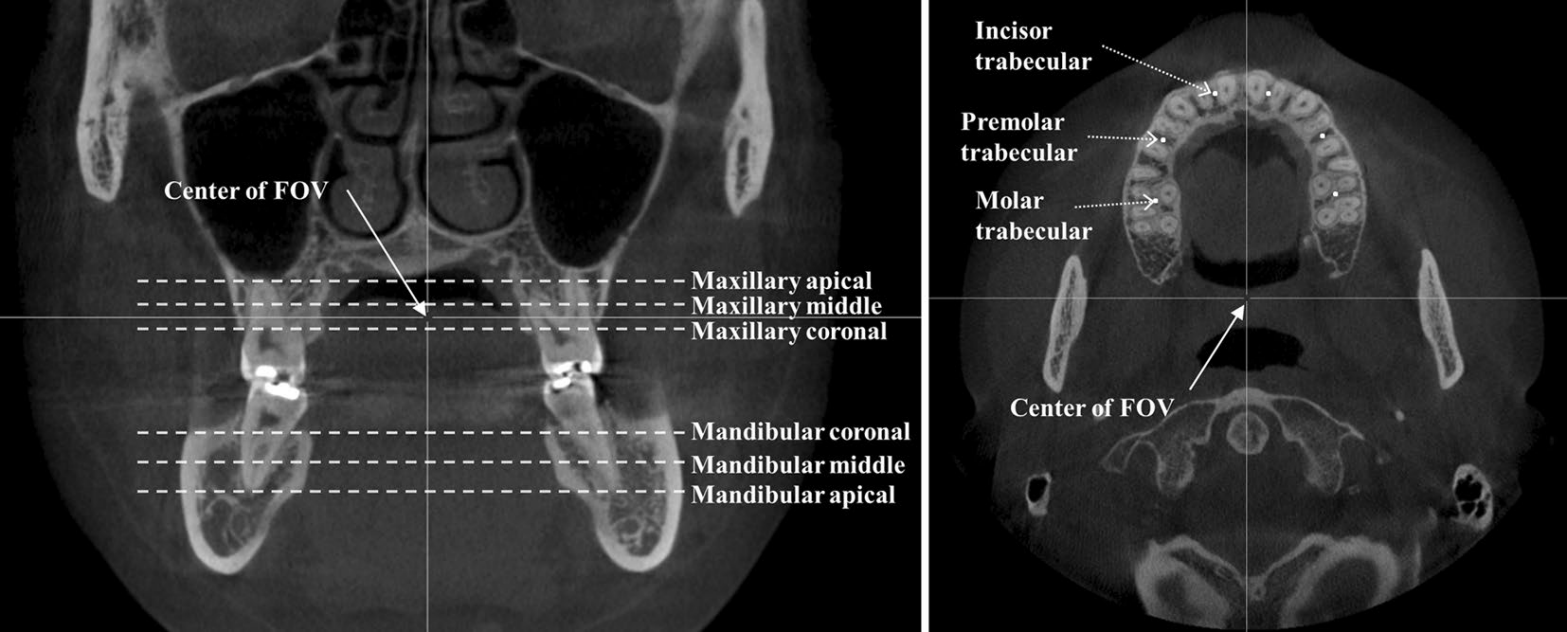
Bone sites of interest at the maxilla and mandible across the entire field of view (FOV) of CBCT. (Left) Axial slices in the coronal, middle, and apical planes along the z-axis in the maxilla and the mandible. (Right) Interdental trabecular bone sites in the left and right incisors, premolars, and molars in same axial plane. The center of the FOV of each CBCT image is indicated by an arrow.
